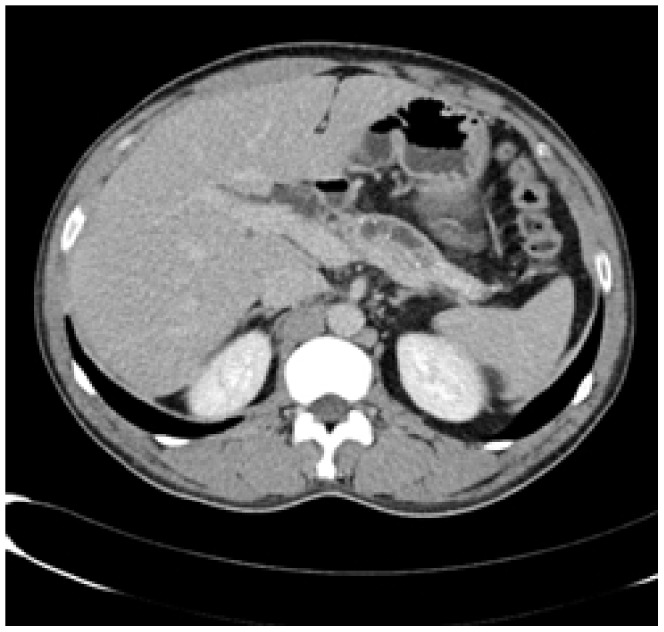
Enhanced CT revealing dilation and string-of-beads change of pancreatic duct,calcification or pancreatic calculus.
